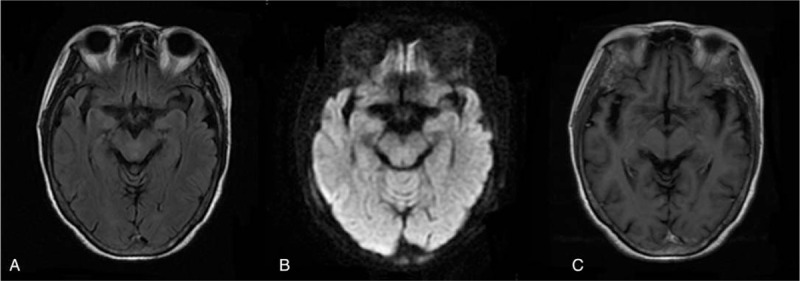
MRI of brainstem showing no abnormal signs. (A) T2-weighted flair image. (B) Diffusion-weighted image. (C) T1 contrast-enhanced image. MRI = magnetic resonance imaging.
